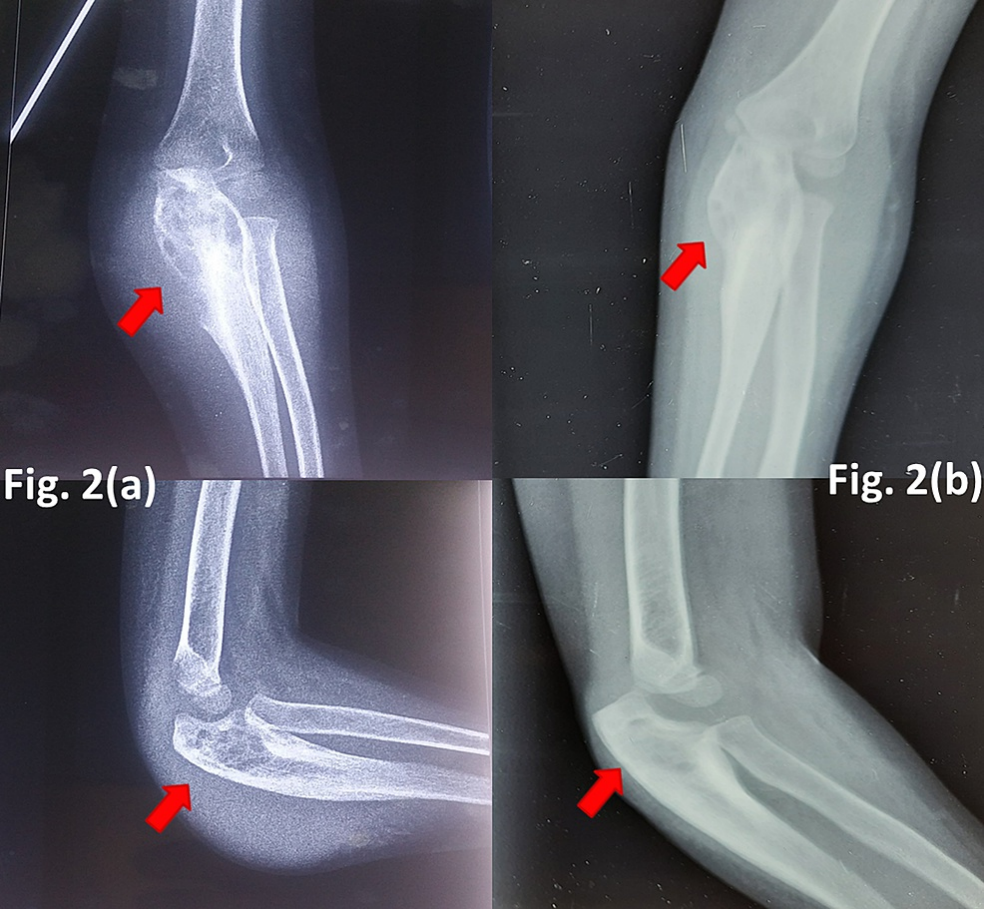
TB of the olecranon process of ulna (a) Plain radiograph at presentation (b) At 15 months follow-up TB - tuberculosis
